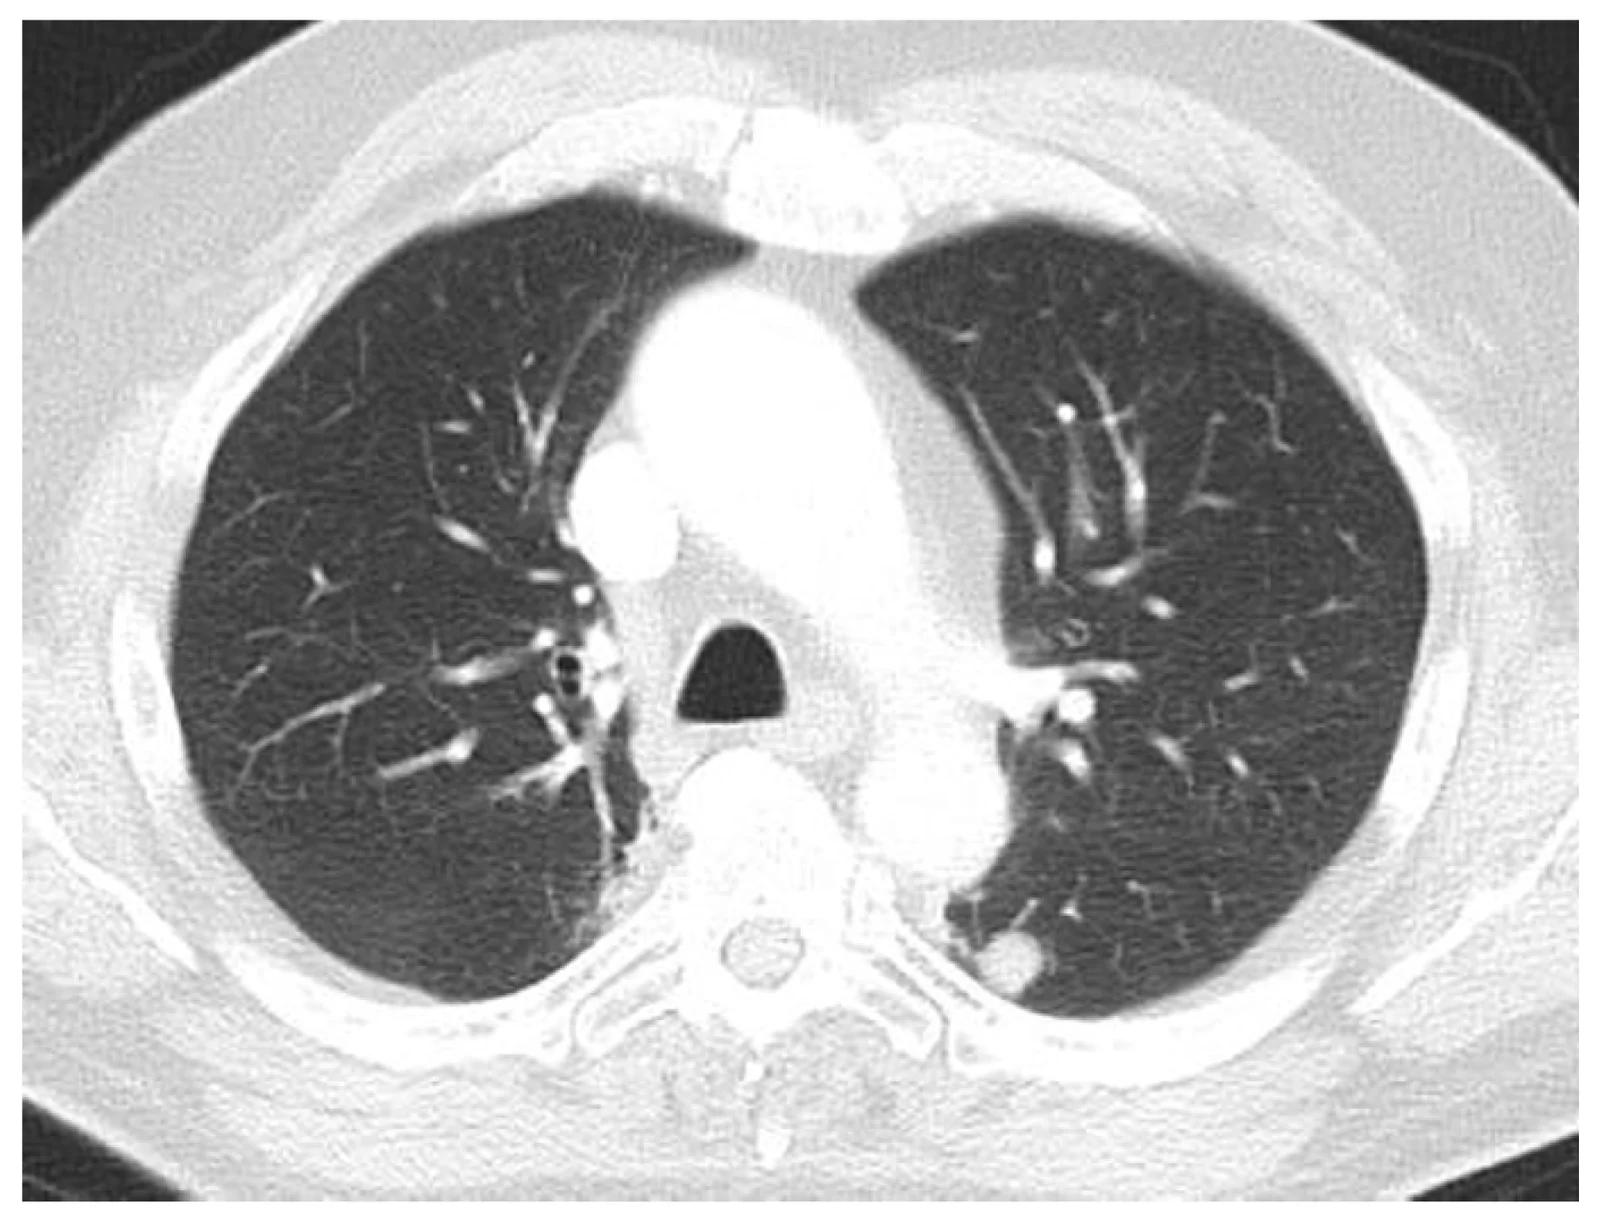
Axial chest CT showing a 1.2 cm nodule in the left lower lobe of the lung.
